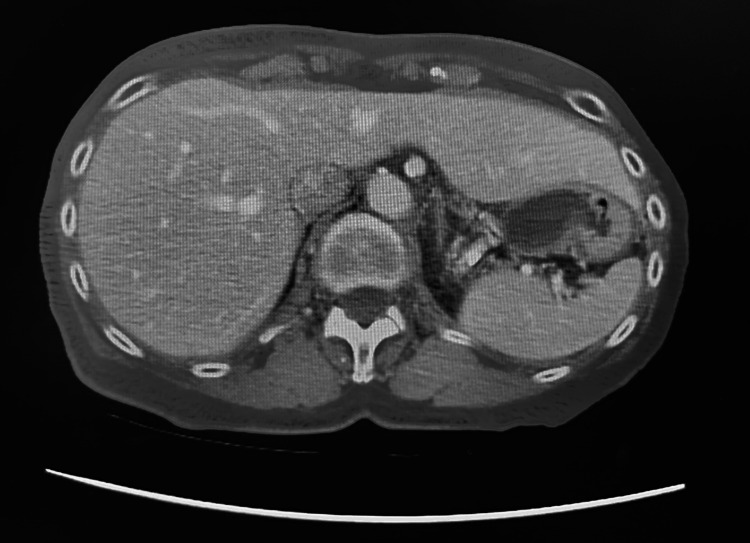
CT showing empty gallbladder fossa
